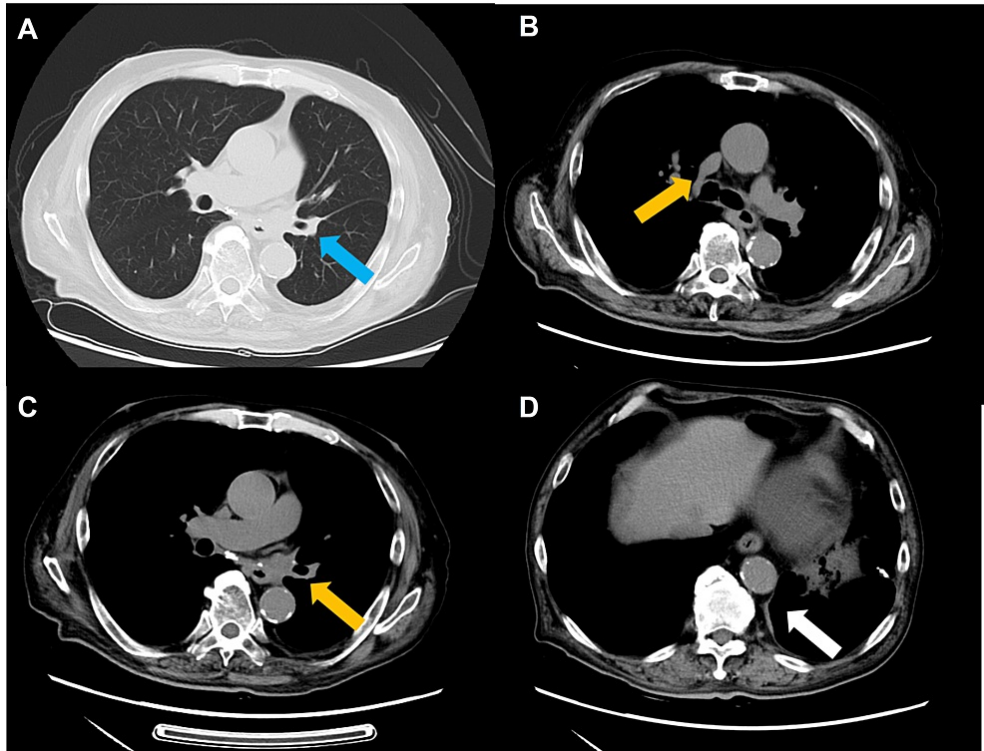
Chest computed tomography at the onset of fulminant type 1 diabetes mellitus. Chest computed tomography at the onset of fulminant type 1 diabetes mellitus demonstrated (A) shrunk primary lesion (blue arrow), (B and C) lymph nodes (yellow arrow), and (D) pleural dissemination (white arrow). A complete response was achieved.
